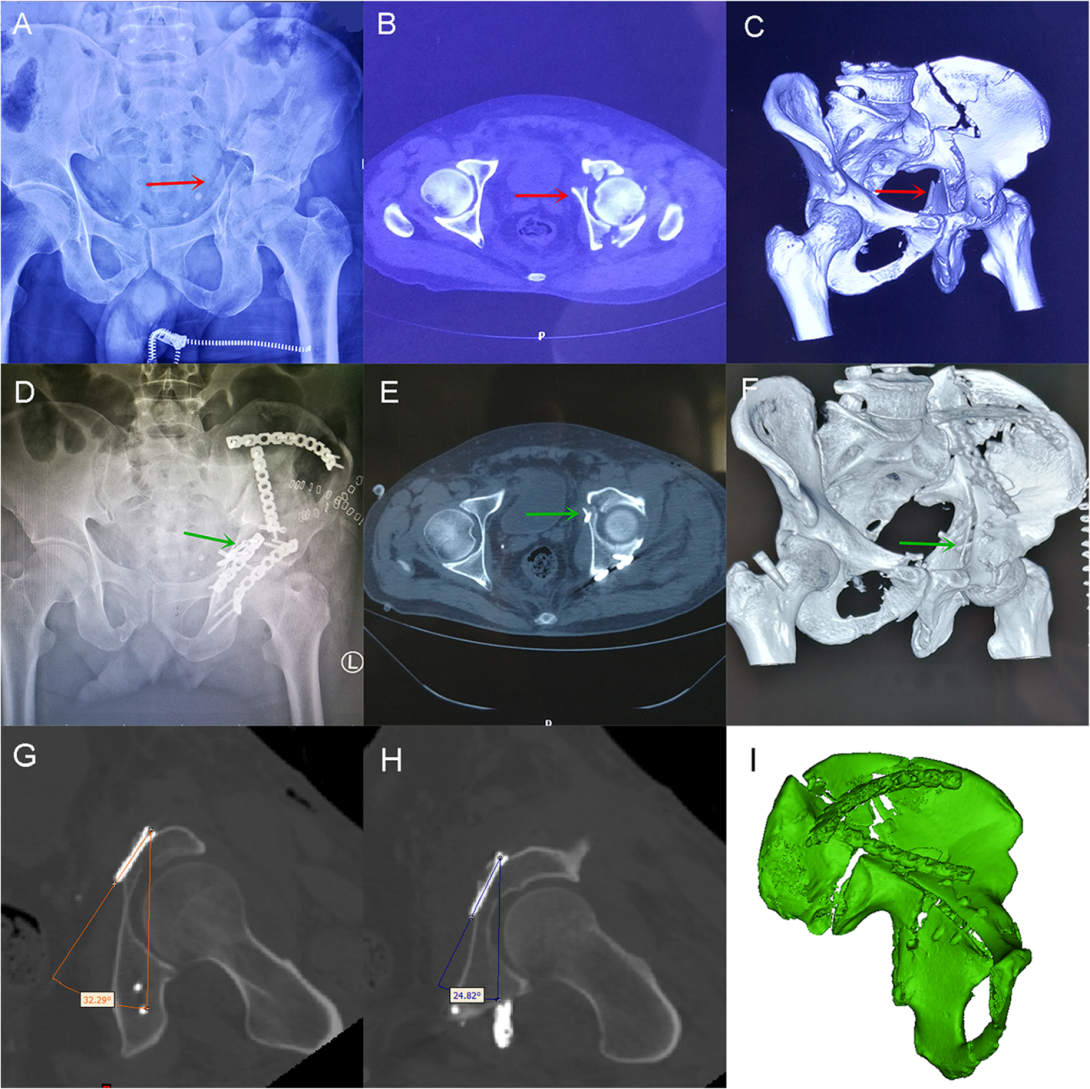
A 51-year-old man was suffered serious traffic injuries. a-c: preoperative X-Ray, CT cross section and 3D reconstruction. The red arrow represents the displaced quadrilateral plate fracture. d-f: postoperative X-Ray, CT cross section and 3D reconstruction. Green arrow shows two hollow countersunk screws used to fix the quadrilateral plate. g-i: Postoperative CT-scan images were imported into Mimics software. Observation of the coronal plane and 3D model of the semi-pelvis shows that the insertion angle of the screws corresponded to that of our study
